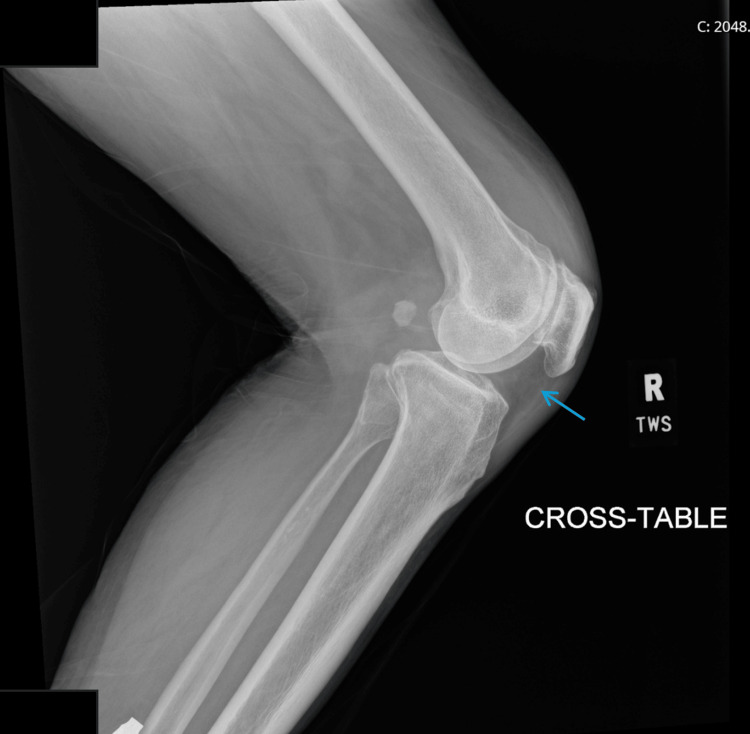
Initial X-ray in the emergency department showing a joint effusion
